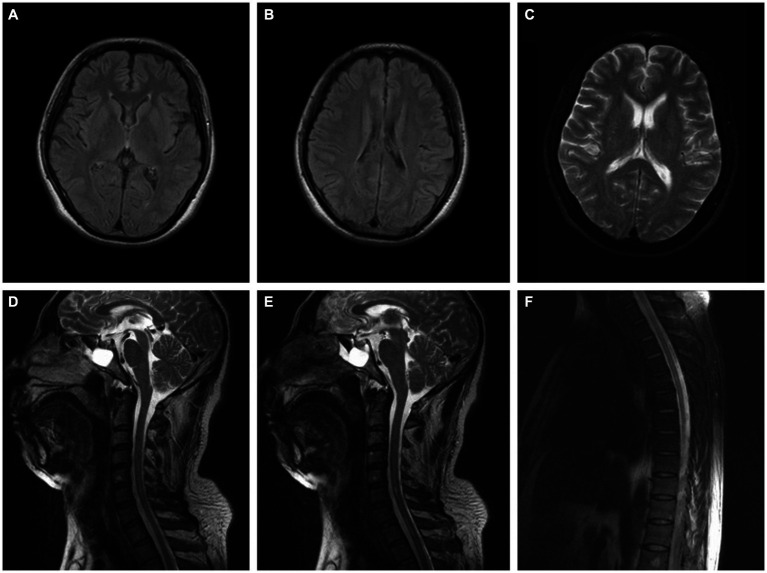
(A–C) Brain MRI of the patient in case 2 was normal (2 months after onset). (D–F) Spinal MRI of the patient in case 2 indicated longitudinal linear abnormity with long T2 signal in the cervical and thoracic spinal cord and was not enhanced on the contrast-enhanced scan (2 months after onset).
